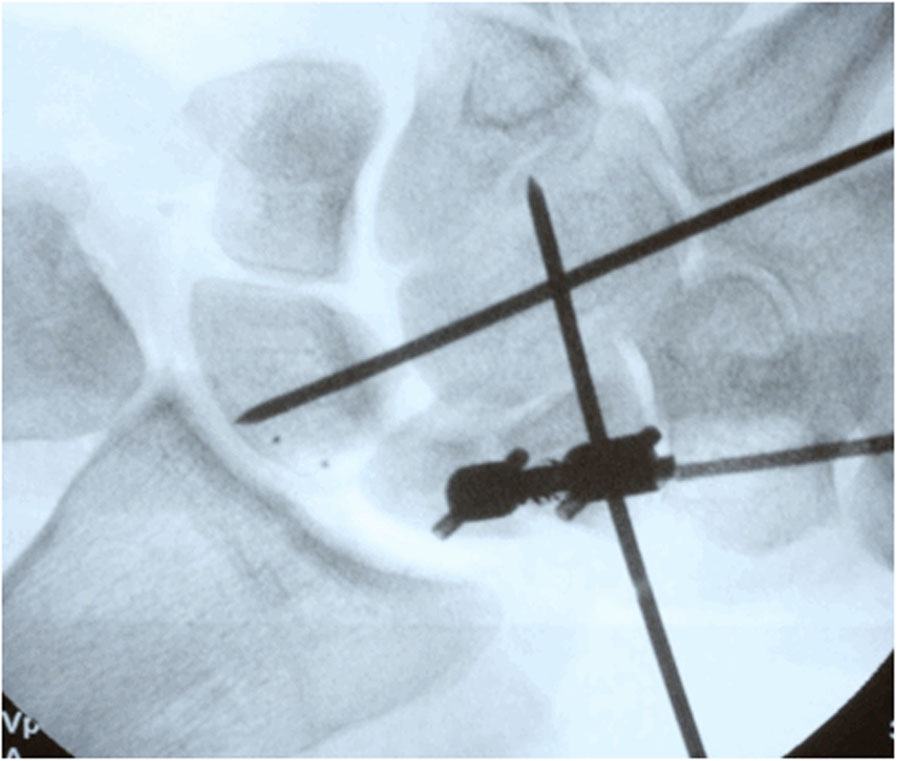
X-ray of the experimental wrist setup. The fixation locking nail was used as a sensor between the two scaphoid fragments. In addition, the midcarpal joint was partially stabilised with K-wires
